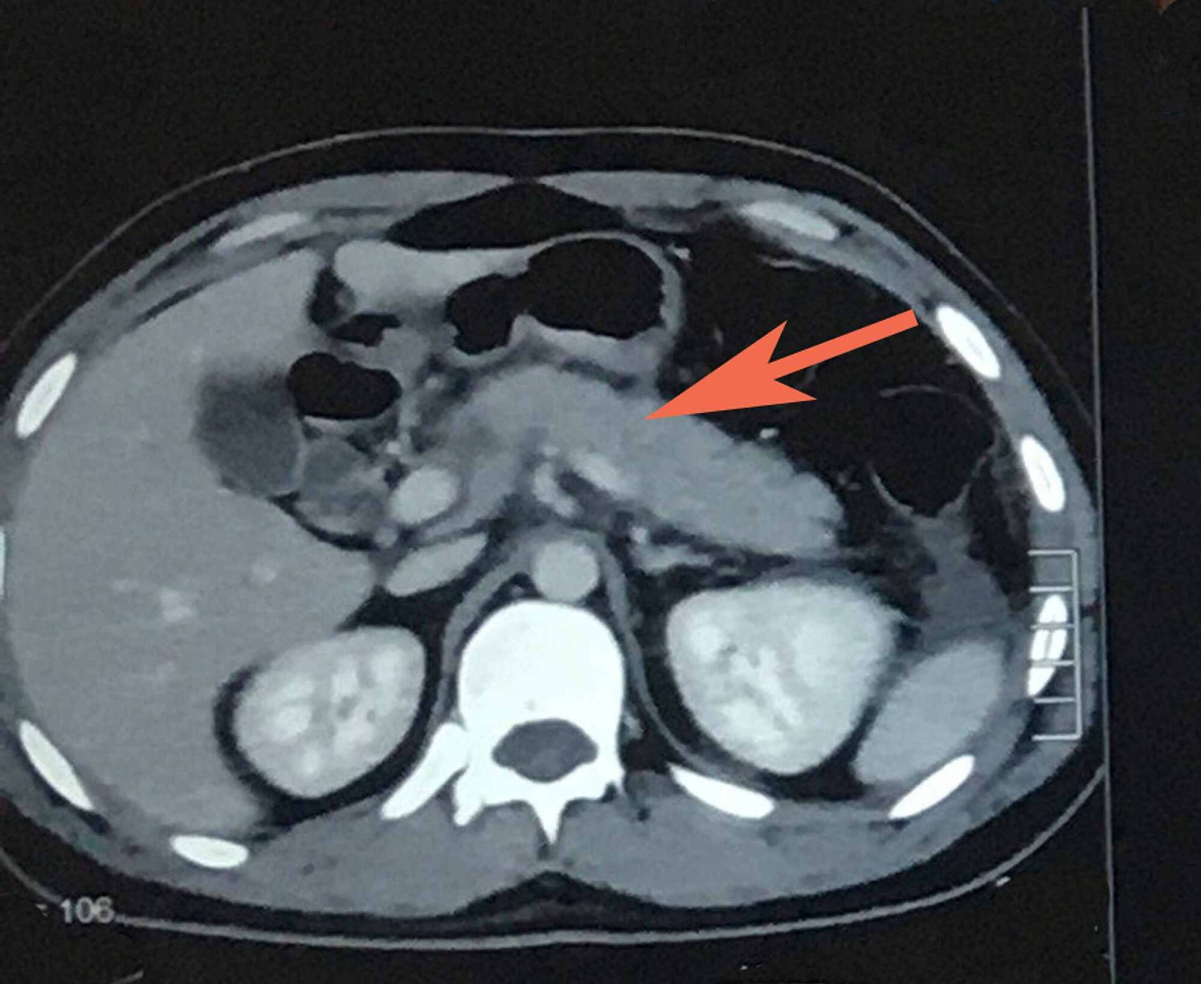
CT abdomen showing pancreatic inflammation highlighted by red arrow, bulky pancreas with peri-pancreatic fat stranding.
